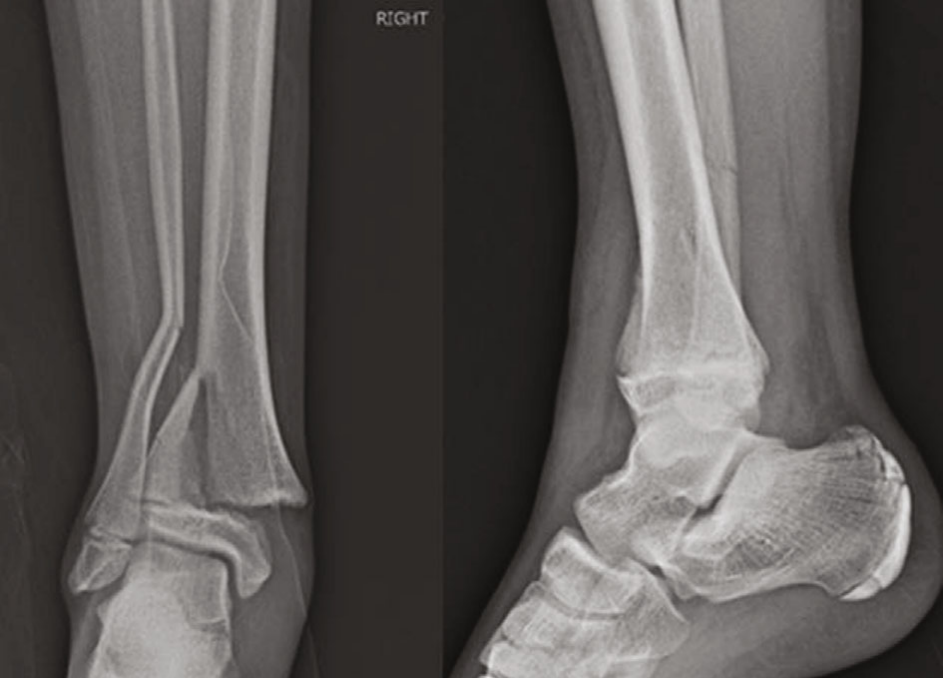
Anteroposterior and lateral radiographs of the ankle fracture at time of presentation.
